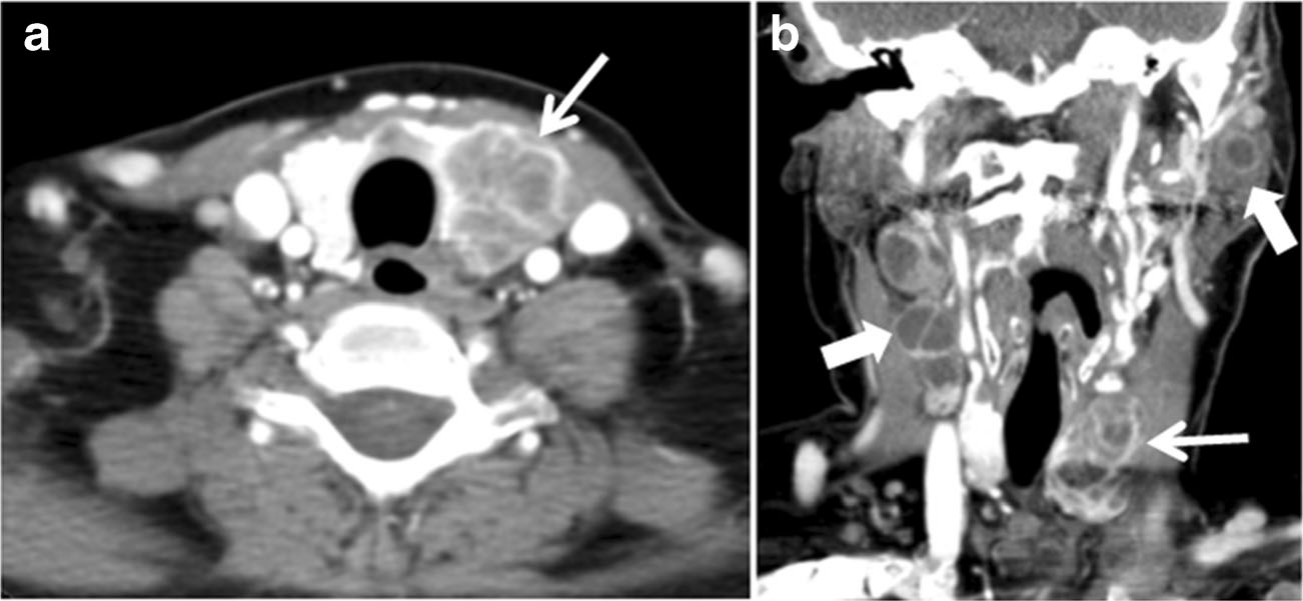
Metastatic squamous cell carcinoma of unknown origin in a 42-year-old female patient. a, b Axial and coronal enhanced neck CT scan demonstrates infiltrative hypodense left thyroid lobe lesions (*white arrows*). There are multiple necrotic cervical nodal metastases (*white block arrows*)
